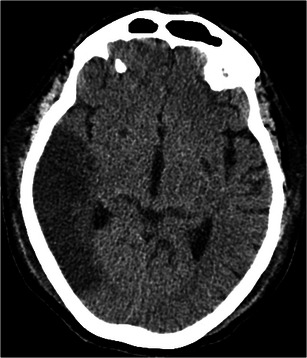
Stroke in a 48-year-old male on VA ECMO with mental status change. CT of the head demonstrates a large hypodensity in the distribution of the right MCA compatible with subacute infarct
